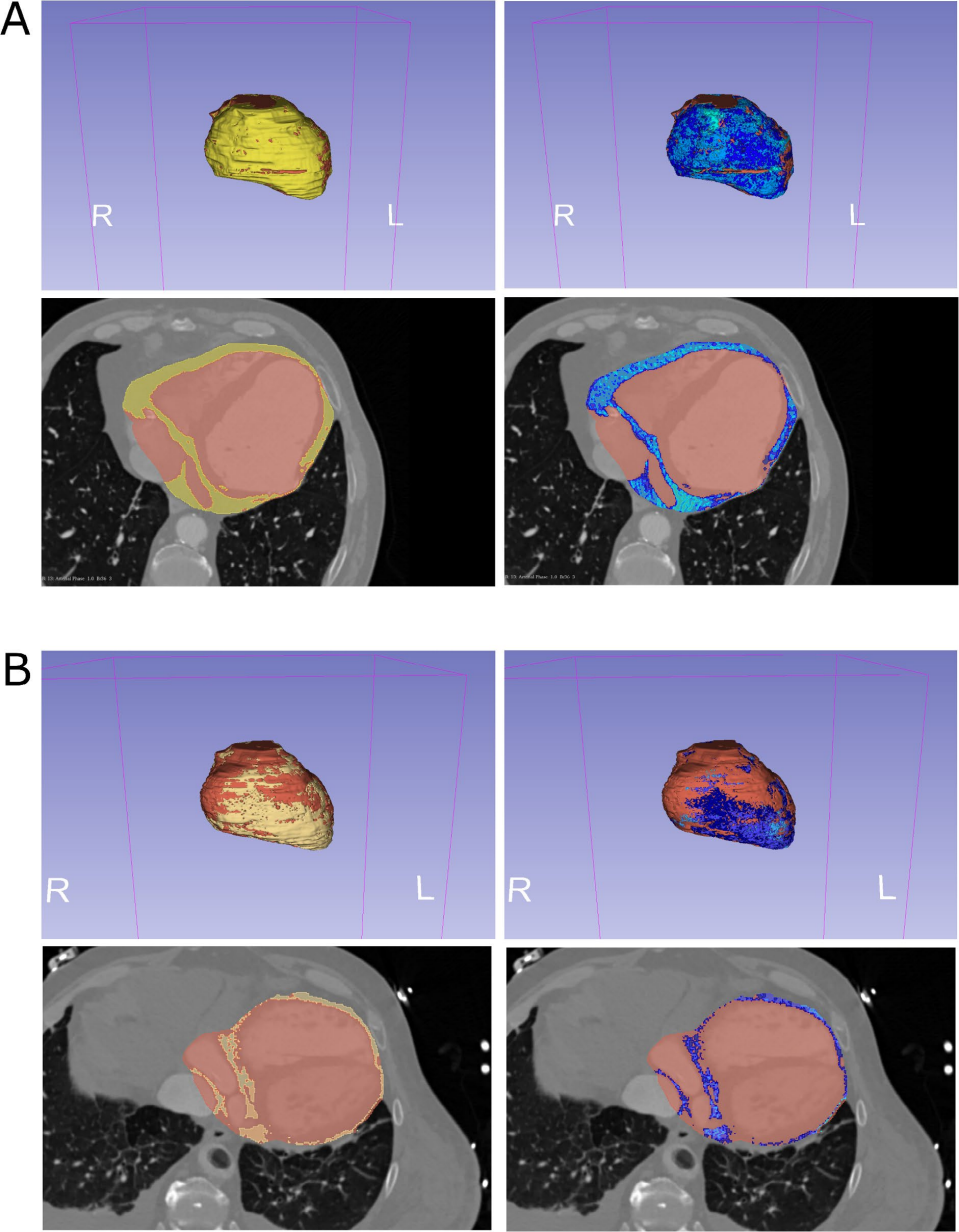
Computed tomography imaging of epicardial fat. Epicardial adipose tissue (EAT) volume and density analysis in contrast-enhanced computed tomography (CT) scan of the chest using open-source software 3D Slicer (https://www.slicer.org/) (our unpublished data). Left panels - red color indicates pixels within the space limited by the visceral layer of the pericardium (traced manually), whereas yellow color indicates EAT (detected using density threshold from -30 HU to -180 HU). Right panels - EAT density gradients: light blue - the lowest (-180 to -120), through blue (-120 to -90) to dark blue (-90 to -30). Upper panels - 3D reconstructions, lower panels - a single transverse slice. [A] Patient 1 with a thick layer of EAT, with low EAT density. [B] Patient 2 with a thin layer of EAT and high EAT density
